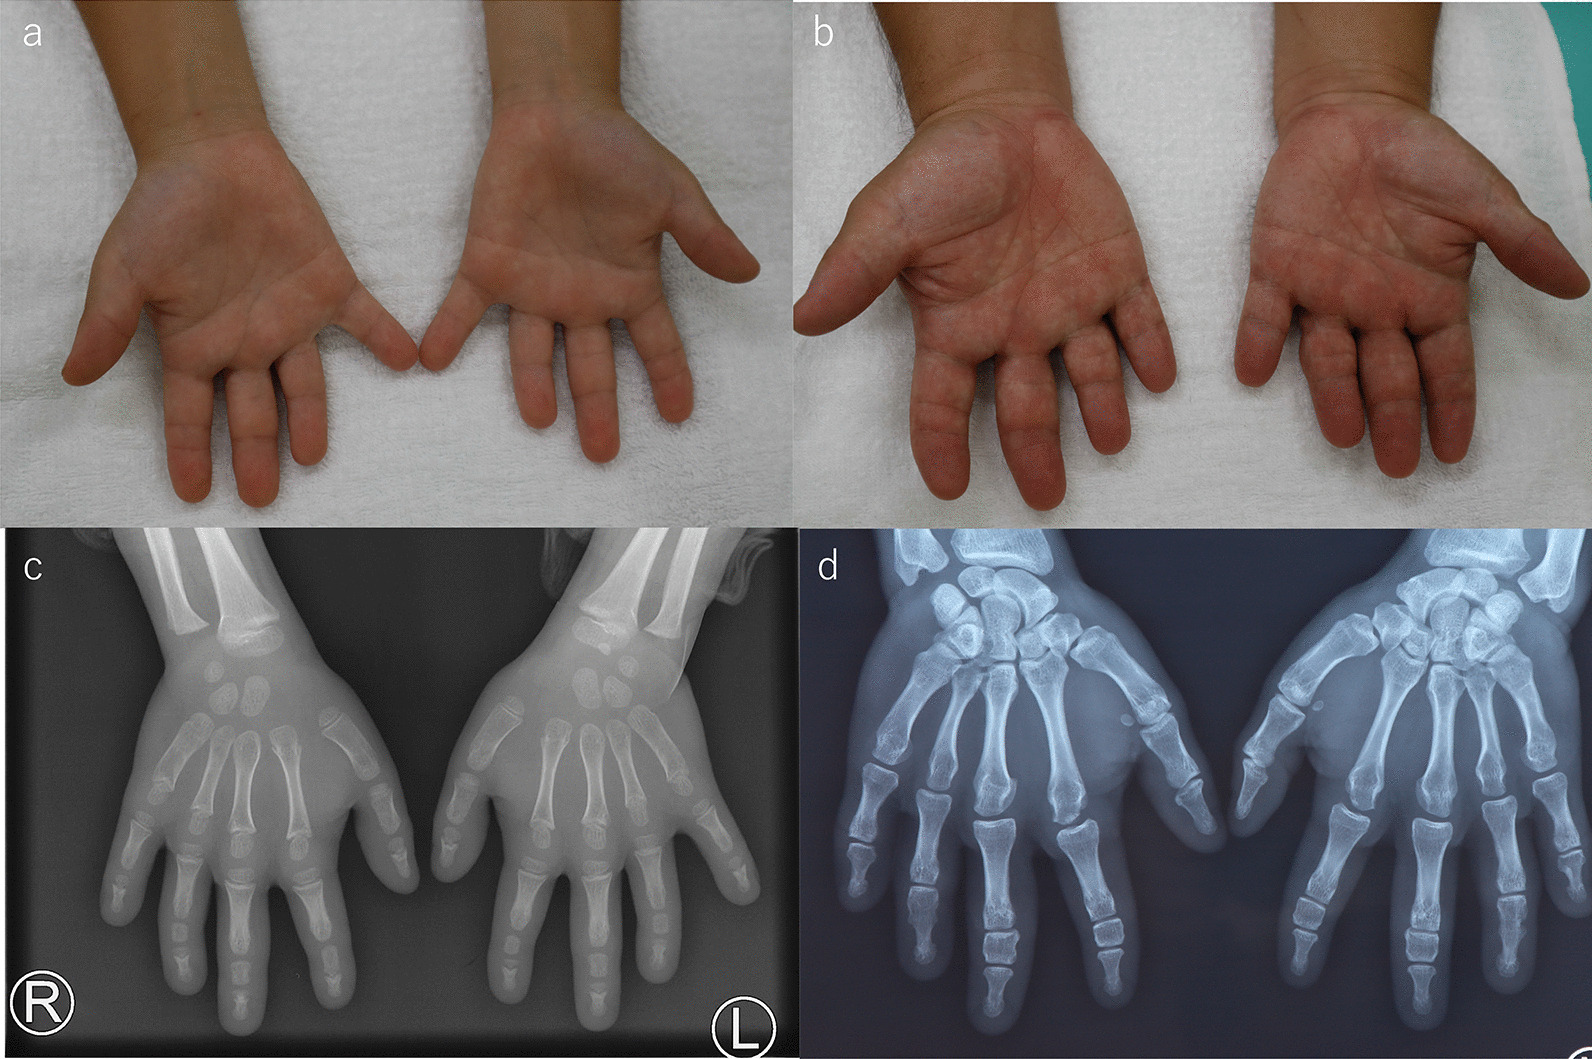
a–d The appearance and radiographic findings of the proband and his father with BDA1. a, b Proband and his father, respectively, showing shortening of all digits, missing interphalangeal creases in the third to fifth digits and mild clinodactyly of the fourth digit. c Proband showing shortening of the middle phalanges of the second to fifth digits and fusion of the middle and terminal phalanges of the fifth digit. d Father showing severe shortening of the middle phalanges of the second and third digits, and fusion of the middle to the terminal phalanges of the fourth and fifth digits
